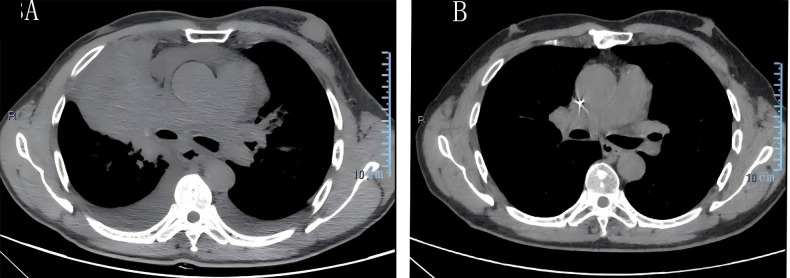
Computed tomography (CT) images of the breast lesion. (A) Initial detection of the breast lesion in August 2023. (B) The lesion had completely resolved by January 16, 2026.
